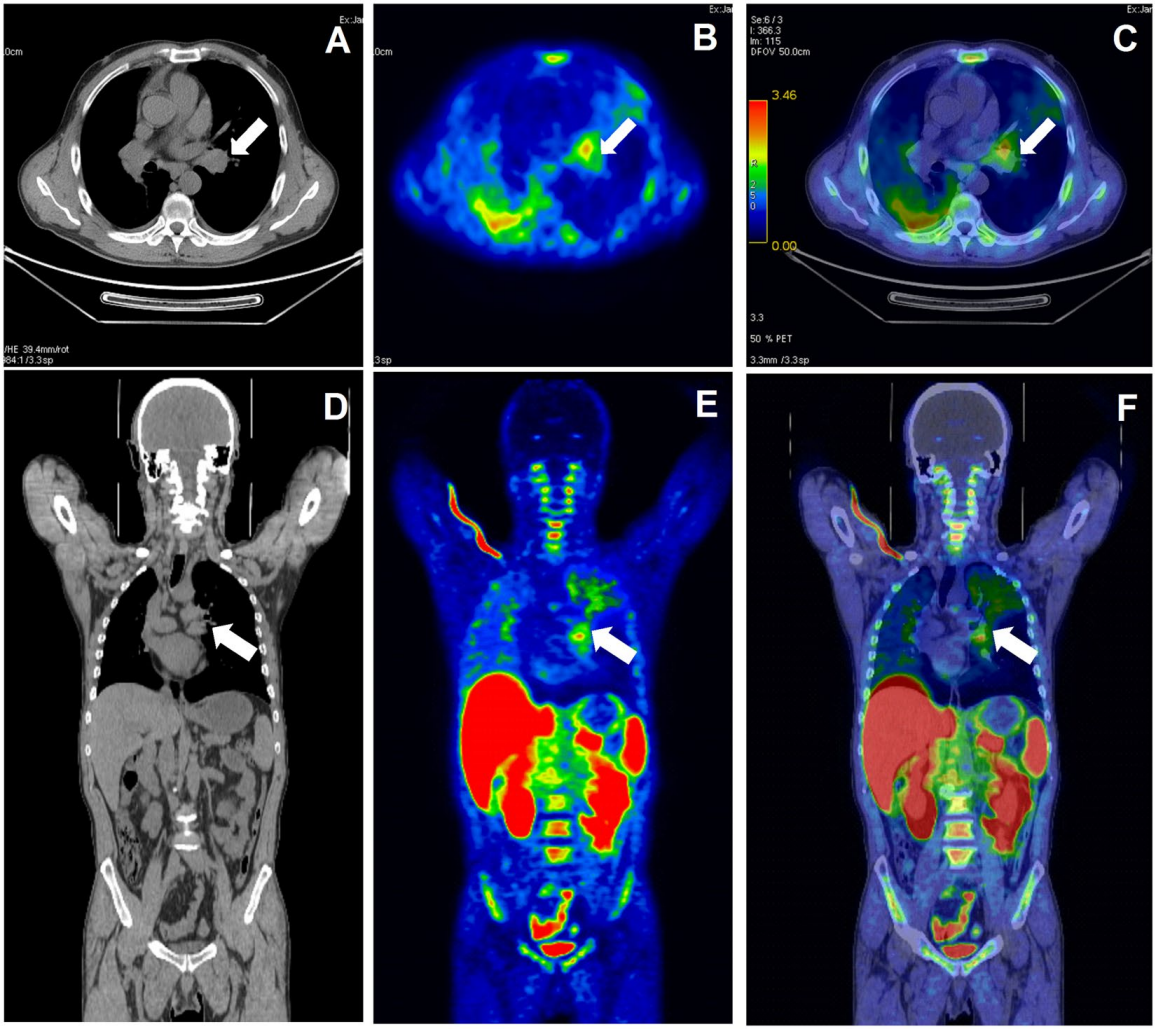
18F-IRS PET/CT scan in a patient with NSCLC of left lung. (white arrow indicates tumor area) (A) Transaxial CT image. (B) Transaxial PET image. (C) Transaxial PET/ CT image. (D) Coronal CT image. (E) Coronal PET image. (F) Coronal PET/CT image.
